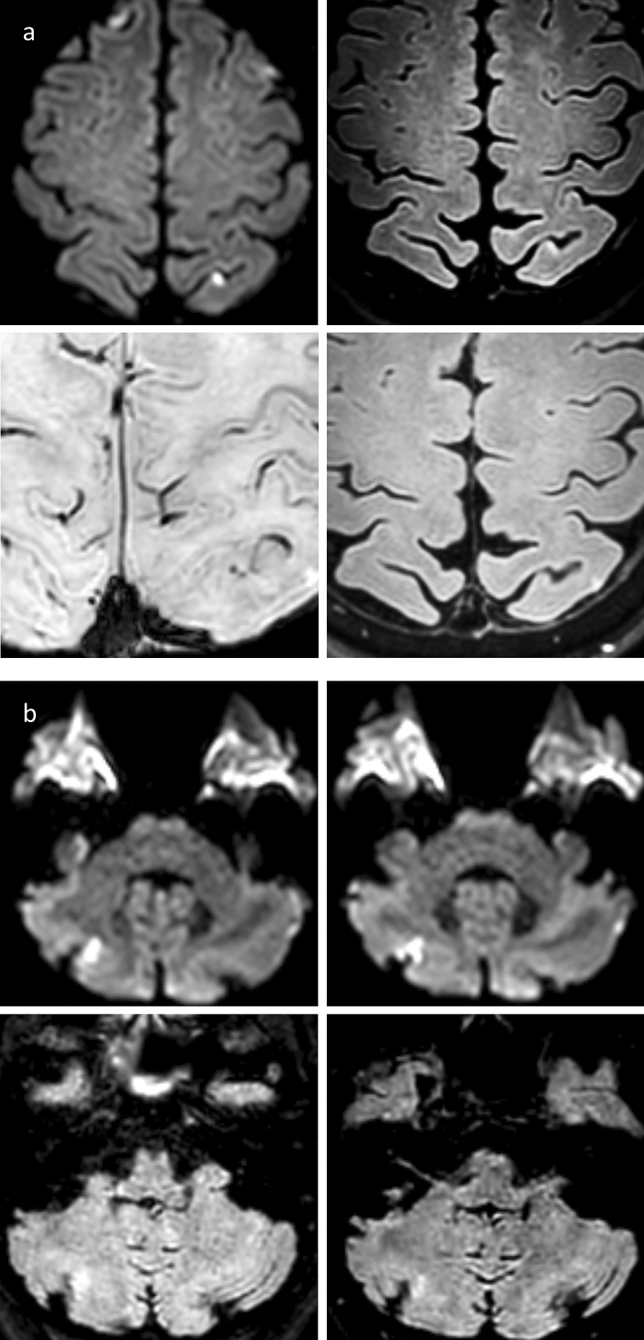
Persistent high-signal lesions at follow-up. a Subject No. 24. Upper row shows DWI (left) and slightly zoomed-in FLAIR (right) after DSA. Bottom row shows zoomed-in FLAIR (right) and even more zoomed-in SWI (left) at follow-up. DWI after DSA shows high signal corresponding to diffusion restriction in left superior parietal lobule. Diffusion-restricted area shows slight FLAIR hyperintensity following DSA and at follow-up with punctate low-signal in SWI. b Subject No. 45. Upper row shows DWI at different heights after DSA with a high-signal diffusion-restricted cortical lesion of the right cerebellar lobe. Bottom row shows FLAIR after DSA (left) and at follow-up (right) with well visible persistent FLAIR hyperintensity at both time appointments. Abbreviations: FLAIR, fluid attenuated inversion recovery; DSA, digital subtraction analysis; DWI, diffusion-weighted imaging; No, number; SWI, susceptibility-weighted imaging
